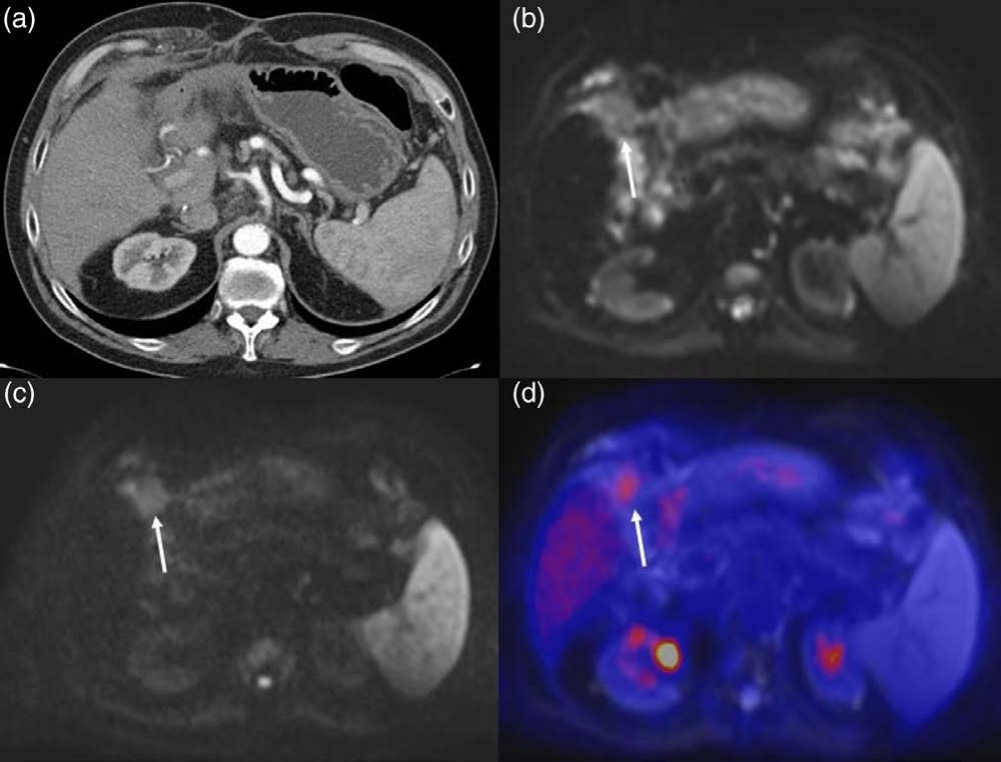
In a patient with persistently elevated alpha-fetoprotein levels, contrast-enhanced CT was not able to identify any recurrences (a), while PET/MRI detected an area of signal restriction (arrow) in the S4b segment of the liver (b,c), showing radiotracer uptake (arrow) at PET/MR fused images with a SUV_max_ of 4.9 (Fig. 3b–d). At biopsy, the lesion was confirmed to be an hepatocellular carcinoma (HCC) recurrence. SUV, standardized uptake value.
